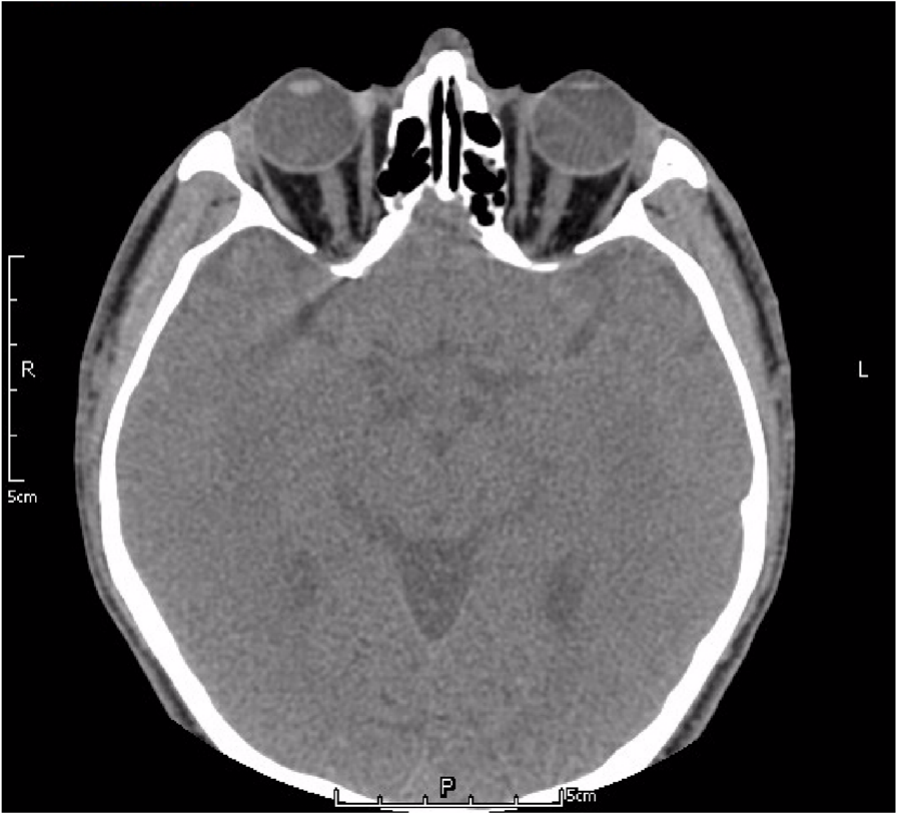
No intraocular foreign bodies (IOFBs) were identified on 0.625-mm thin-sliced helical CT
